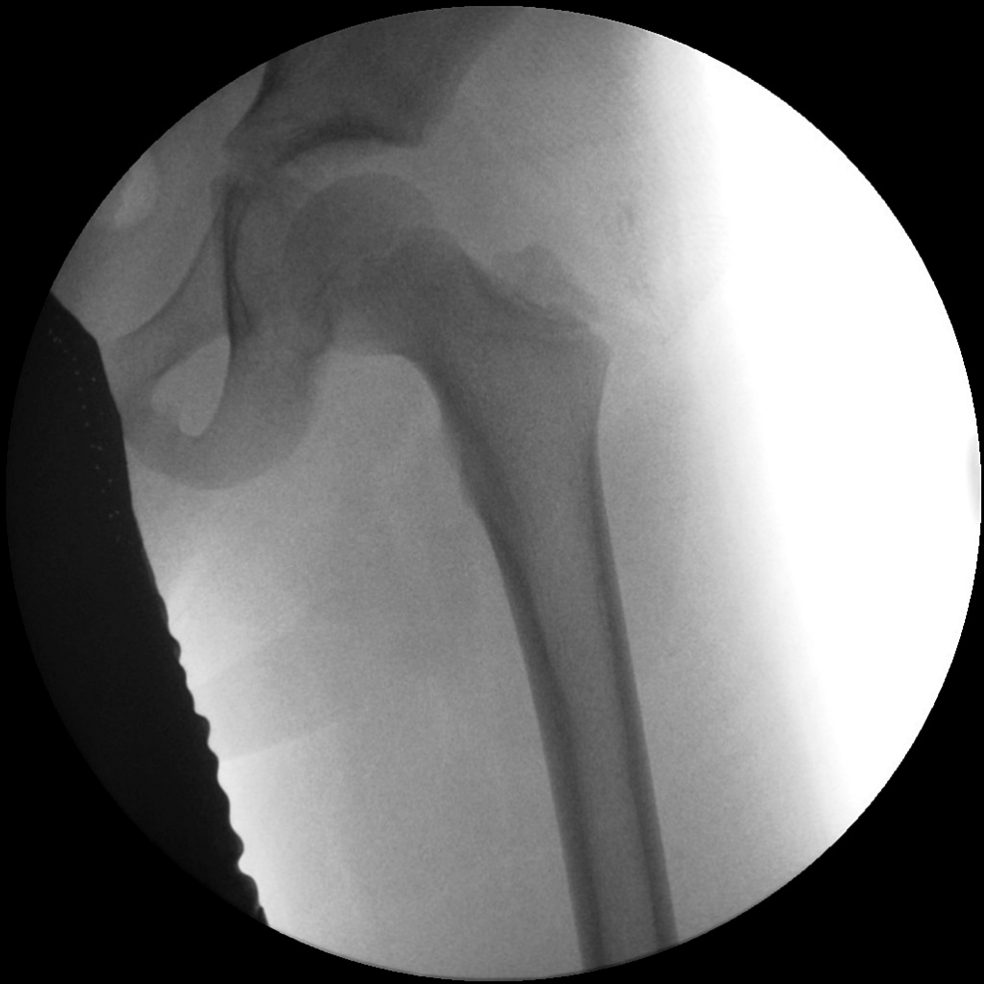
Post reduction X-ray confirming the concentric reduction of the left hip.
